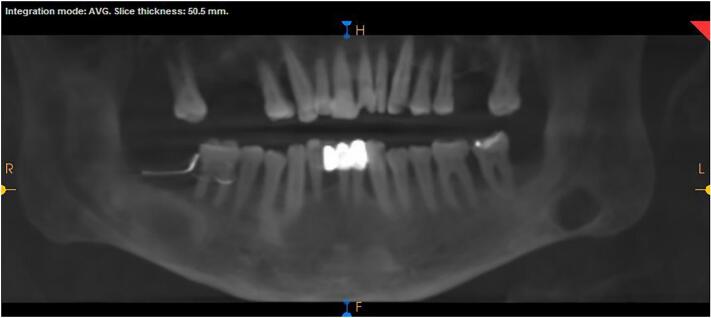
Follow up radiograph after 10 months showing decreasing in the cystic size with bony filling and the decompression tube.
